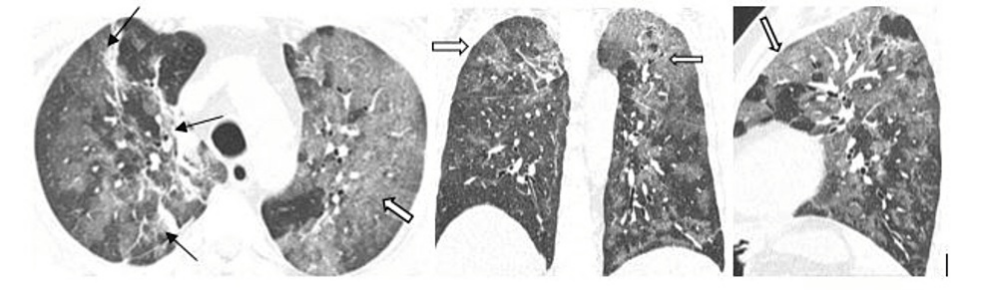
HRCT axial, coronal, and sagittal images of 56Y/M Covid-19 positive patient showing multilobar of patchy ground-glass opacities (block arrow) in bilateral lung parenchyma, crazy paving in left upper lobe, subsegmental atelectasis and parenchymal bands (arrow) in right upper lobe.
